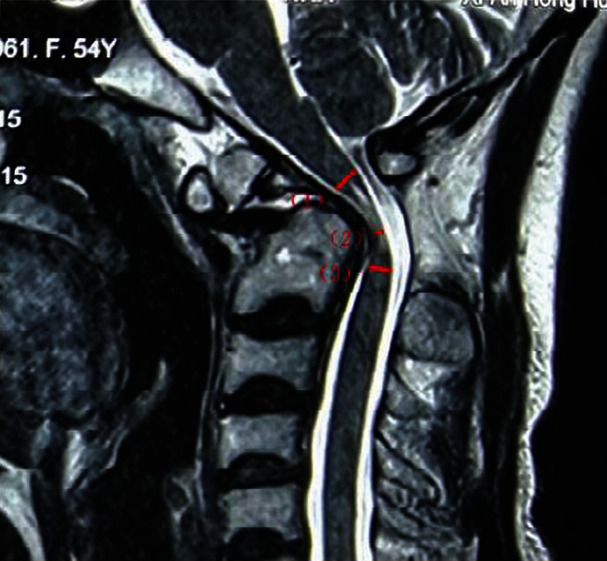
Sagittal neutral MRI showing an example of measurement of SCC: (1) normal spinal cord diameter above SCC; (2) narrowest spinal cord diameter; (3) normal spinal cord diameter below SCC. SCC: spinal cord compression.
